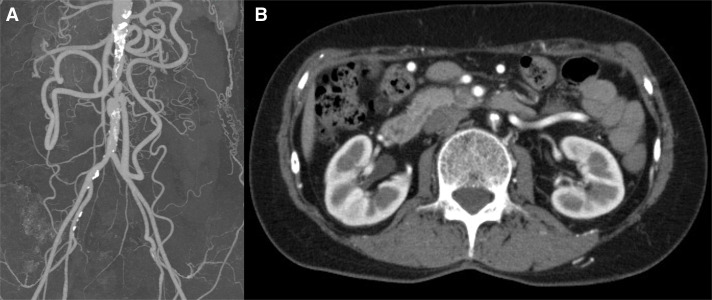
Preprocedural CTA. (A) CTA showing severe stenosis of the aorta between the renal arteries and the IMA, and well-developed collateral flow to the distal abdominal aorta. (B) Axial image of the stenosis in the infrarenal abdominal aorta. IMA, inferior mesenteric artery
